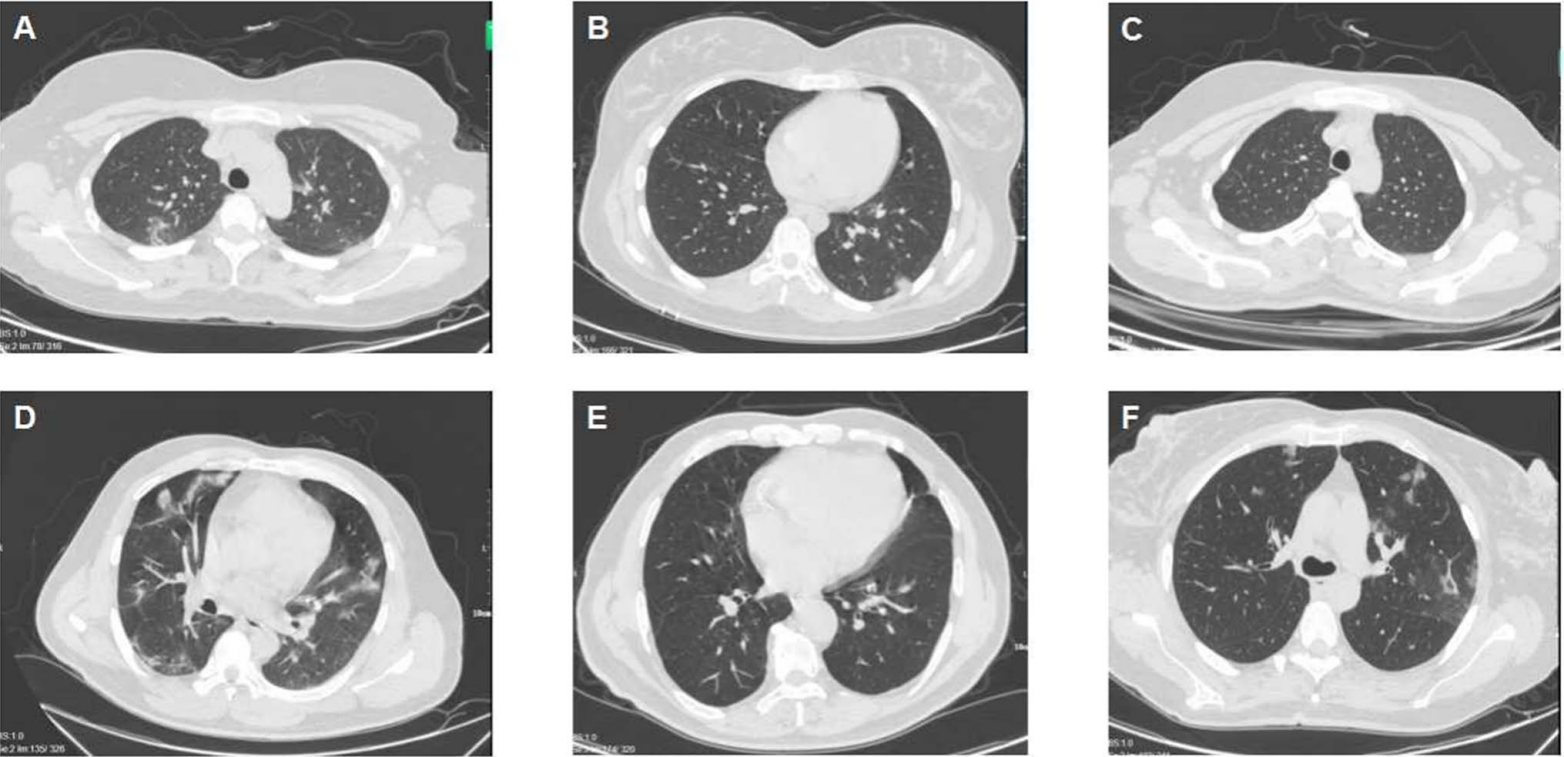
Chest computed tomography scans of six patients with SARS-CoV-2 infection in Inner Mongolia during the Spring Festival of 2022. A Case 1: Mild interstitial changes are present in the lower lobe of both lungs, along with multiple ground-glass opacities; B Case 2: Multiple ground-glass opacities are present in the lower lobe of both lungs, along with ground-glass nodules in the dorsal segment of the lower lobe of the left lung; C Case 3: Solid nodules are visible in the dorsal segment of the lower lobe of the left lung, along with ground-glass nodules in the posterior segment of the upper lobe of the left lung; D Case 4: Multiple patchy, nodular ground-glass density shadows are present in both lungs; E Case 5: Dense shadows of ground-glass opacity and linear opacities are present under the pleura in the lower lobe of both lungs, along with in the basal segment of the lower lobe of the right lung; F Case 6: Multiple patchy consolidation shadows and ground-glass density are present in the posterior apex and lingual segments of the upper lobe of the left lung and the middle lobe of the right lung, calculi are also present in the right kidney
